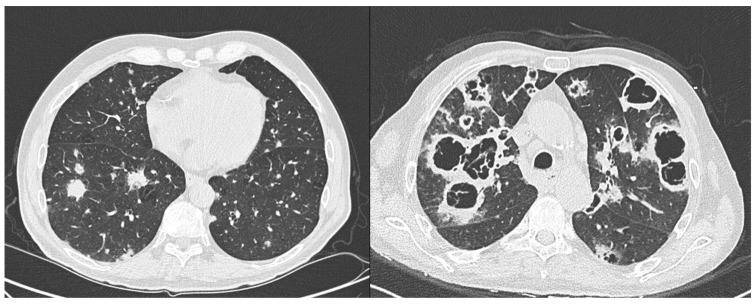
CT chest before and after Mogamulizumab treatment. Previously seen nodular lesions are substituted by massively necrotic and cavitated lesions.
